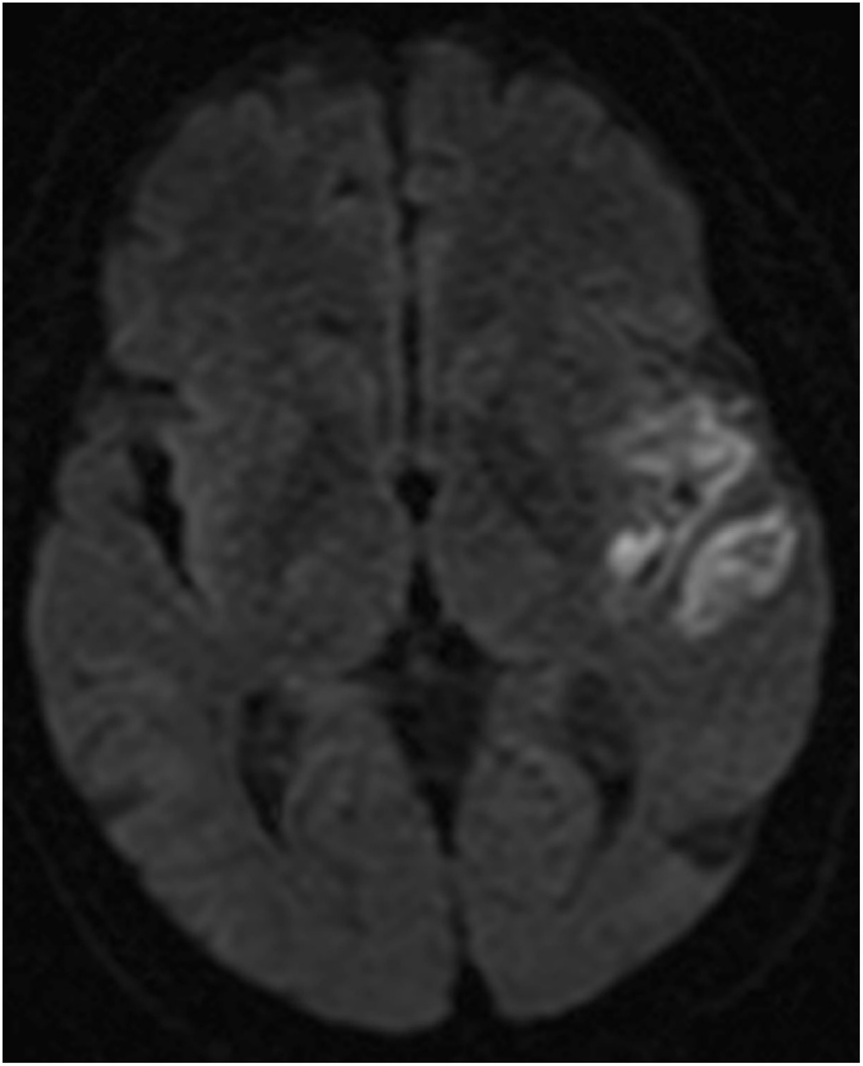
DWI MRI sequence completed once the patient presented with delayed ischemic symptoms. There is evidence of restricted diffusion in DWI with hyperintensity in the ADC map (not shown) in the same left middle cerebral artery vascular territory, indicating early subacute infarction.
